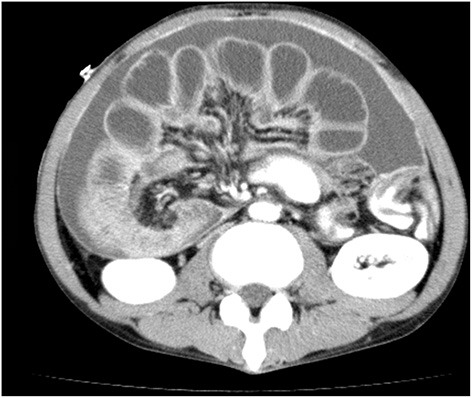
CT findings of cases with highly advanced gastric cancer. Multiple dilations and wall-thickening of the small bowel suggest multiple small-bowel obstructions are found.
